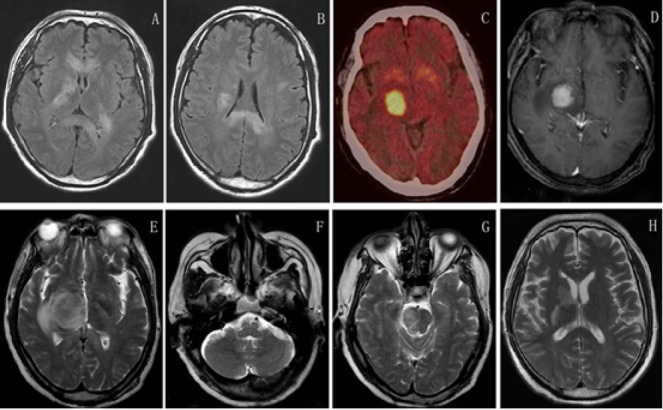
Magnetic resonance imaging revealed that right basal ganglia, pons, cerebellar peduncles with abnormal signals.（April 5^th^） A and B: Magnetic resonance scans showed bilateral thalamus, periventricular, basal ganglia, pedunculus cerebri with hypersignals using fluid attenuated inversion recovery.（May 19^th^）C: Positron emission tomography (PET) showed a slightly high enhancement in the right basal ganglia and right pons, about the size of which is 2.1*2.0*2cm and the maximum SUV value is 23.6. (May 23^th^) D, E, F and G: A month and a half later, axial T1 and T2 magnetic resonance imaging revealed that right basal ganglia, pons, cerebellar peduncles with hypersignals, and lesions were larger than the former, even involved in bilateral thalamus. This enlargement would be suggestive of a neoplastic process. (July 20^th^) H: Axial T2 magnetic resonance imaging revealed that right basal ganglia, pons, cerebellar peduncles with abnormal signal, compared with 2016-05-23, lesions decreased.
